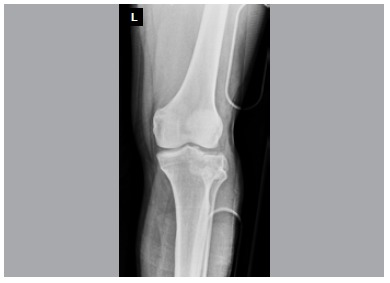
Male, 47 years, preoperative AP X-ray.
